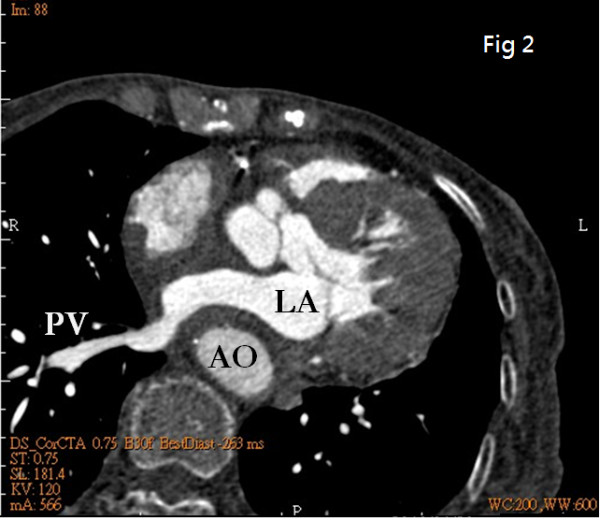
CT images: a displaced descending aorta (AO) constricting the left atrium (LA) is revealed.
